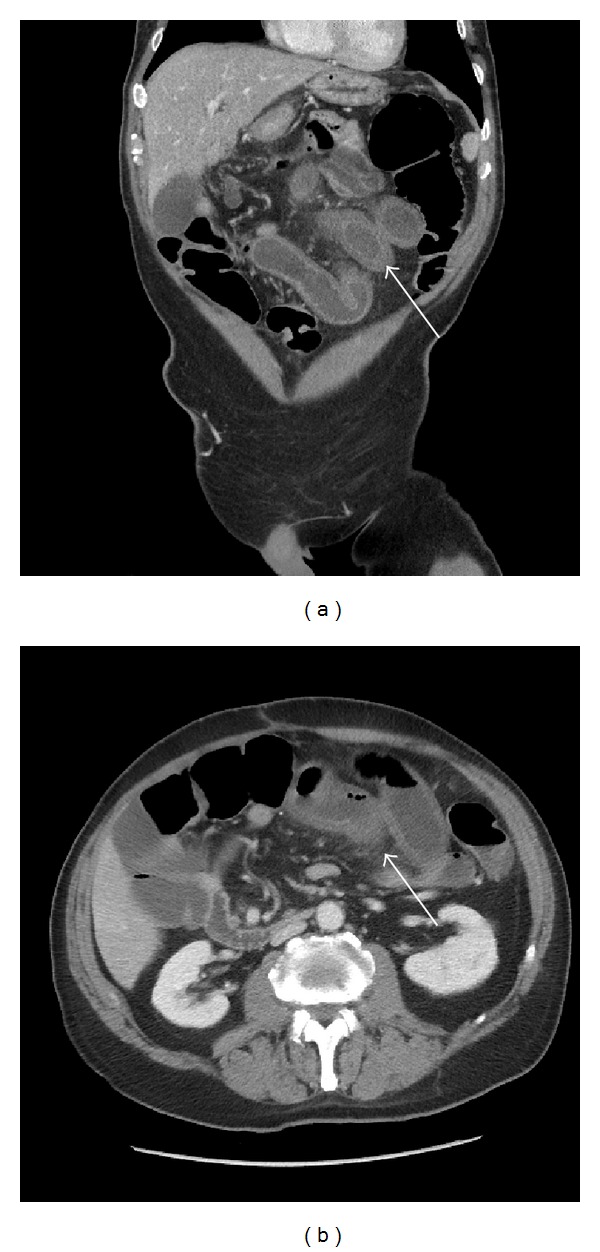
(a) A coronal reformatted image of the CT abdomen and pelvis demonstrating multiple dilated, fluid filled small bowel loops, with thickened and enhancing mucosa in keeping with bowel wall oedema (*Arrow*). (b) Axial images again showing thickened oedematous small bowel loops with surrounding inflammatory fat standing (*Arrow*).
